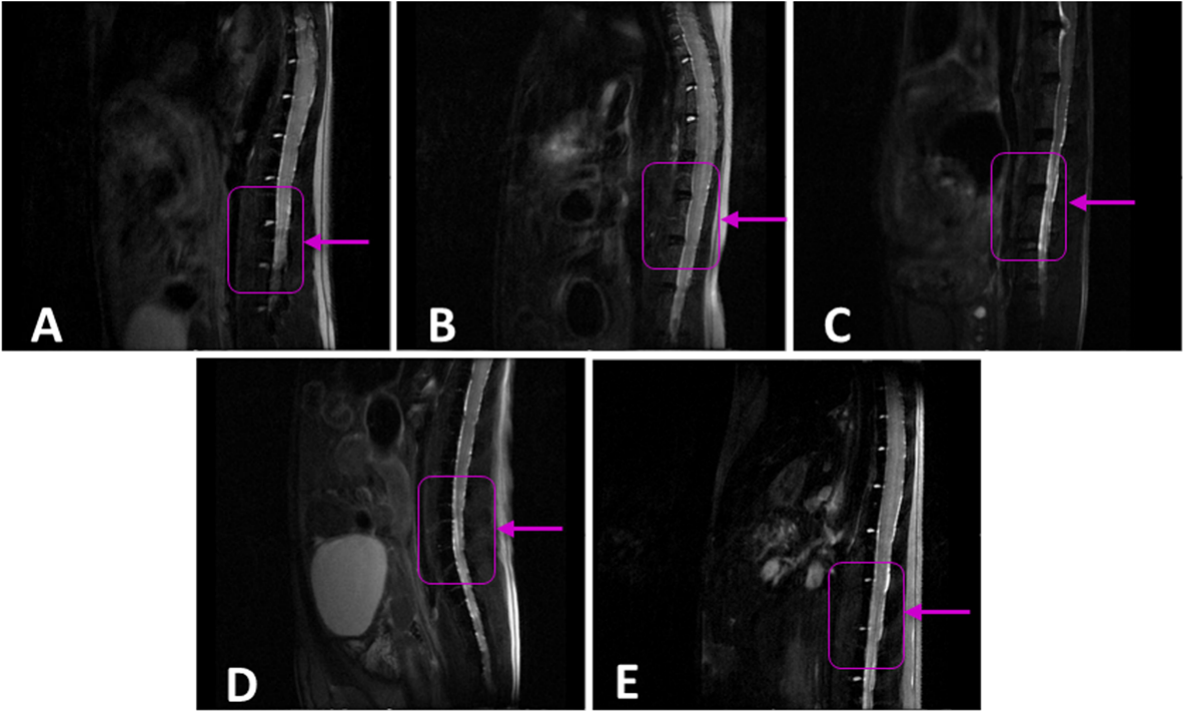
Magnetic resonance imaging characterization of the intervertebral disc (IVD) of biglycan-deficient (
